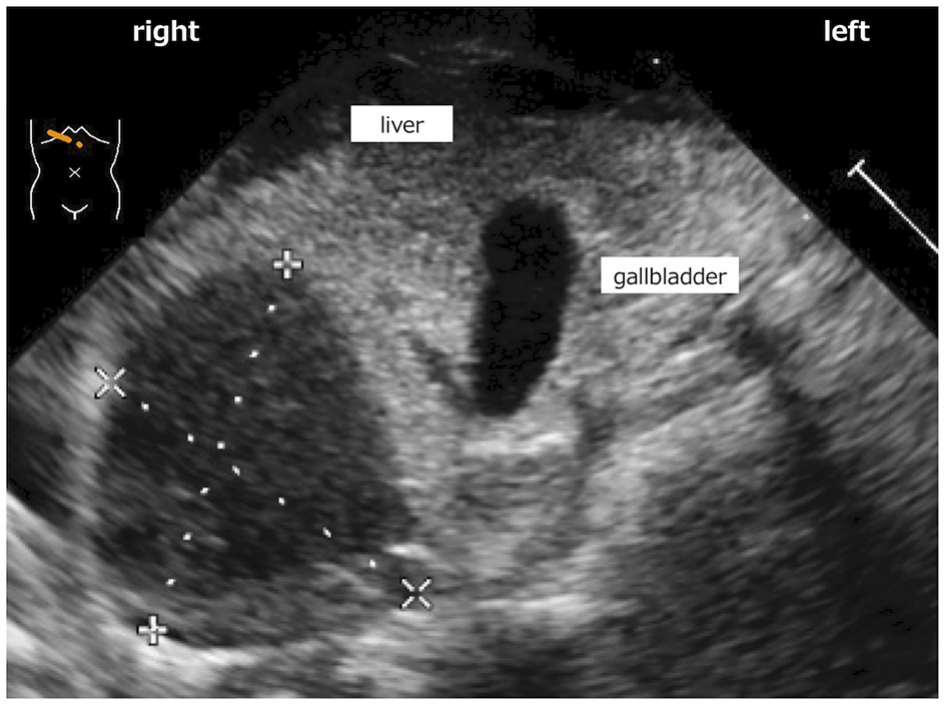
Low echoic lesion in which size was 18 mm × 17 mm that was detected in inferior border of liver on ultrasonography.
